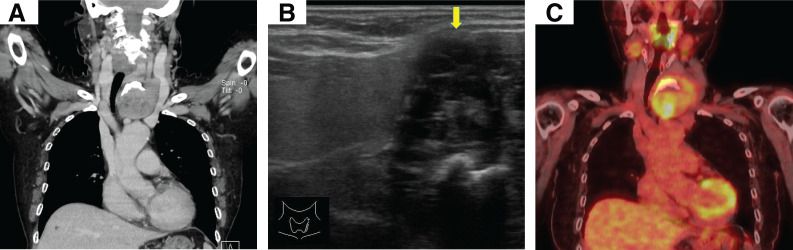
Radiological findings of the tumor. (A) Chest CT shows a 6.5-cm mass inferior to the left thyroid lobe, displacing the trachea to the right. (B) Ultrasonography showing a heterogeneously hypoechoic mass (yellow arrow) with indistinct margins in the left neck that appeared within the left thyroid lobe. (C) Fluorodeoxyglucose PET shows abnormal uptake in the mass.
